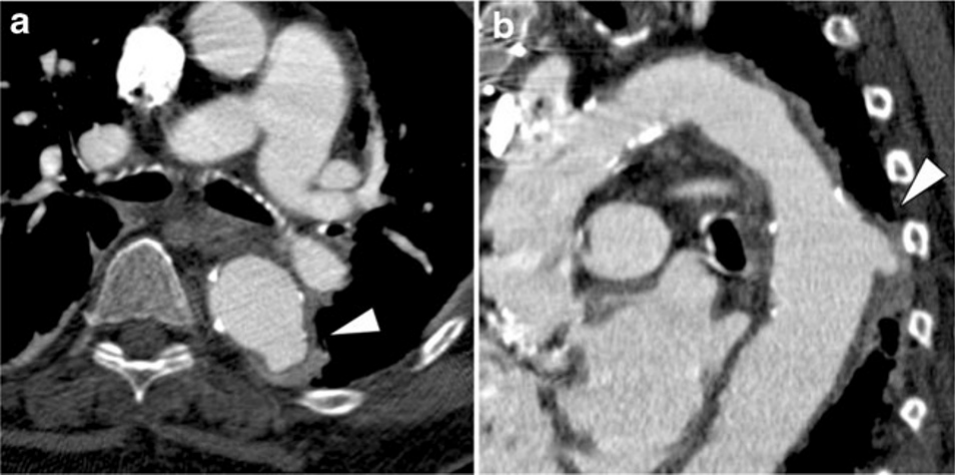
Penetrating atherosclerotic ulcer in an 81-year-old man. a Axial CTA images demonstrate an ulcerative lesion (*arrowhead*) on the thickened aortic wall with haemorrhagic content in the descending aorta. b Oblique sagittal MPR image clearly visualizes a crater-like feature of the ulcerative lesion (*arrowhead*) deeply penetrating into the atherosclerotic aortic wall. Entire aorta depicts severe atherosclerosis with plaque burden and calcifications
